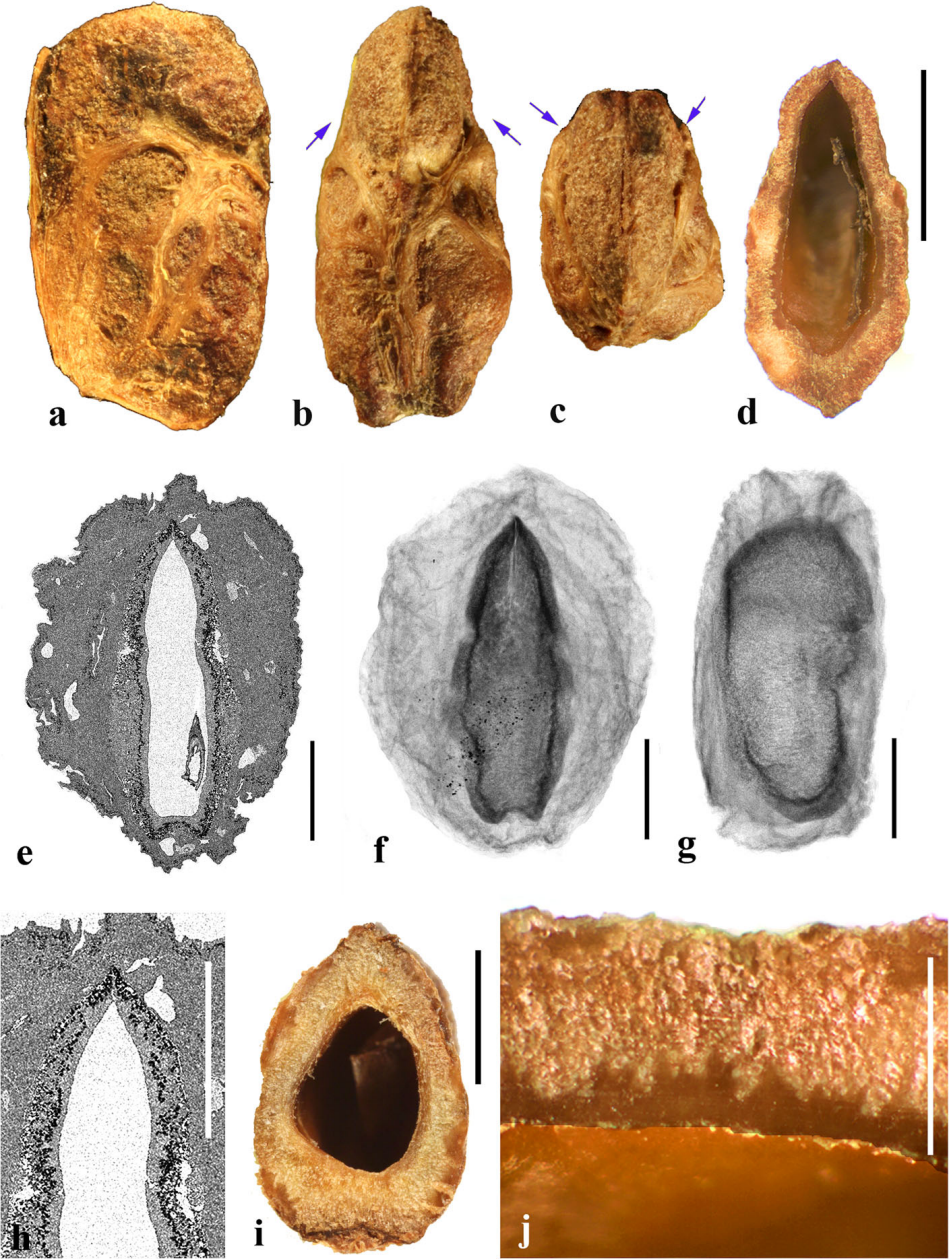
Haplospondias brandisiana* (XTBG: Jianwu Li 847; Yunnan, China). a Endocarp lateral view showing irregular ribs over the lower two thirds of the stone and a smooth, reniform external germination valve. b Frontal view showing the keeled apical edge where the germination flaps meet (arrows). c Apical view; note external flaps (arrows). d Sagittal section of endocarp. e Digital sagittal section of fruit from CT scan data showing thick mesocarp. f, g Coronal and sagittal volume renderings of fruit. h Enlargement from e, showing apically keeled endocarp with vertical line representing separation plane of the apical flaps. i Transverse section of endocarp. j Anatomical detail of locular envelope and endocarp wall. Scale bars 5 mm in d (also applies to a–c); 3 mm in e, h; 2 mm in f, g, i; 0.5 mm in j
